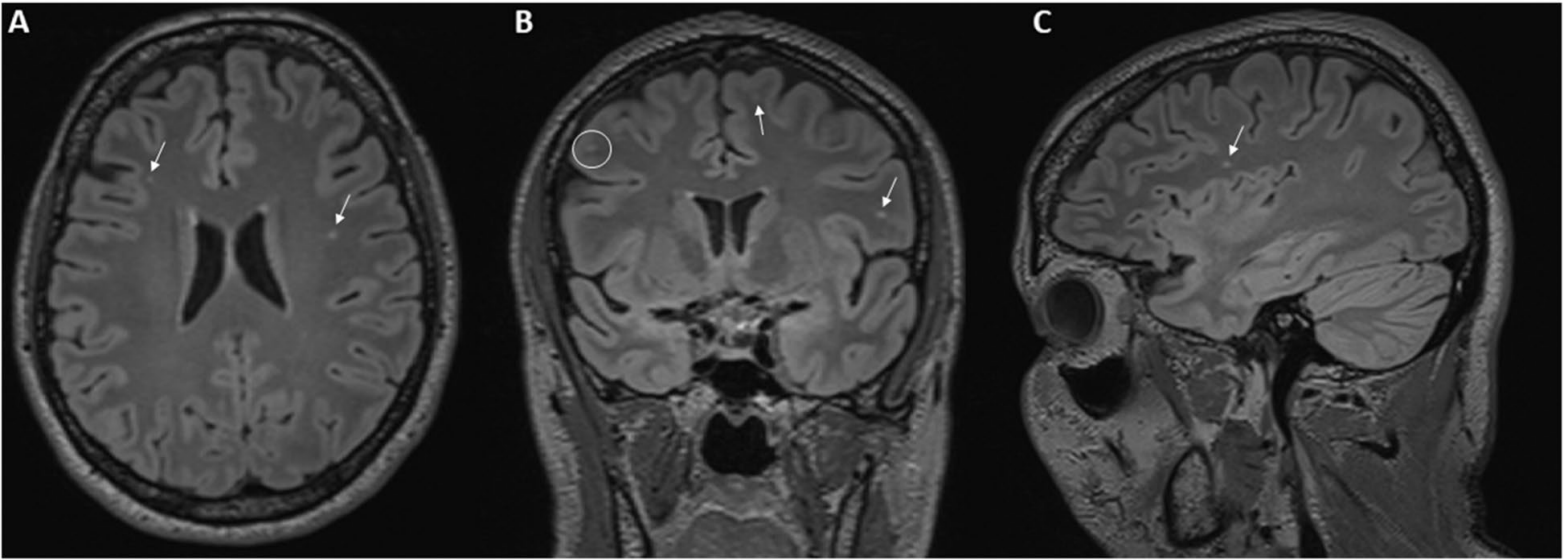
Multiplanar reformats of 3D-FLAIR MRI sequence in a 46 year old lady having migraine over 10 years duration showing different deep white matter hyperintensities (arrows) in axial (A), coronal (B), and sagittal (C) planes. A juxtacortical hyperintensity is also shown abutting the inner margin of the cortex at right middle frontal gyrus (encircled in B)
